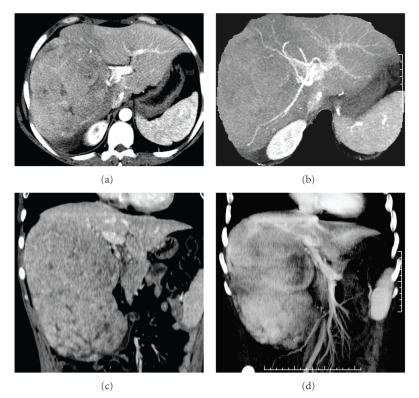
Computed Tomography (CT): axial arterial phase (a); coronal venous phase (c); MIP axial and coronal (b–d). The CT confirmed a mass with heterogeneous density that occupied almost the whole right lobe of the liver (a–c). MIP images showed compressive effects of the mass on sovrahepatic veins; the vessels, were displaced but not infiltrated (b–d).
